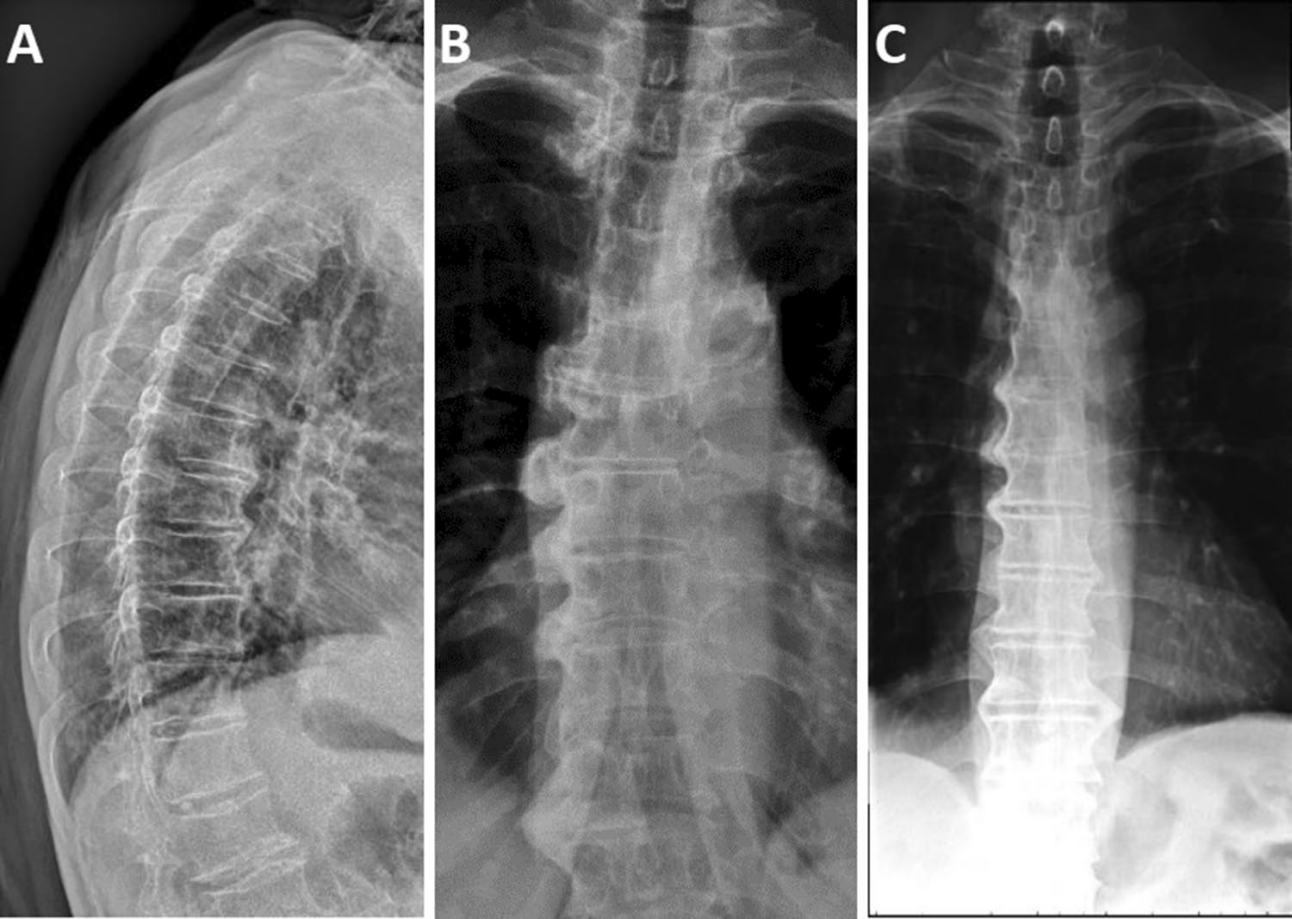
DISH involving different number of vertebrae. a Lateral radiograph showing flowing ossification across 4 vertebral levels; b anterior–posterior radiograph showing bone bridges and large ossification of the anterior lateral ligament spanning 6 vertebral segments; c anterior–posterior radiograph showing flowing ossification involving 8 contiguous vertebral segments on the right side and 4 non-contiguous vertebrae on the left
